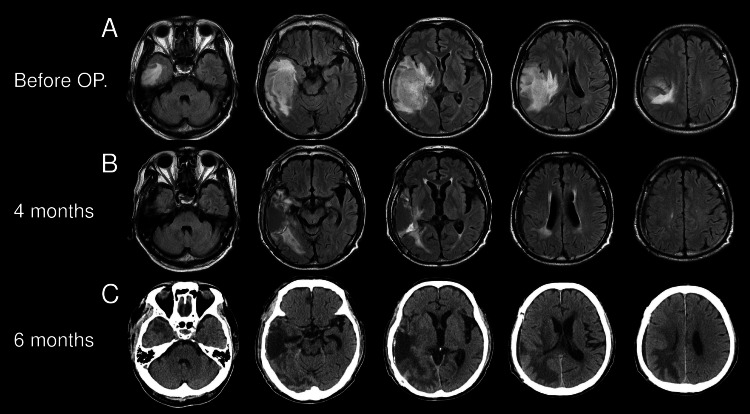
Radiological findings Fluid-attenuated inversion recovery revealed a 40 mm solid tumor with severe brain edema at the right temporoparietal lobe (A). After four months, the fourth maintenance therapy was performed. At this time, the residual tumor and edema were most improved (B). Six months later, the patient complained of headaches and nausea at the start of the sixth maintenance therapy session. The head computed tomography showed severe brain perifocal edema, suggesting regrowth or recurrence (C).
